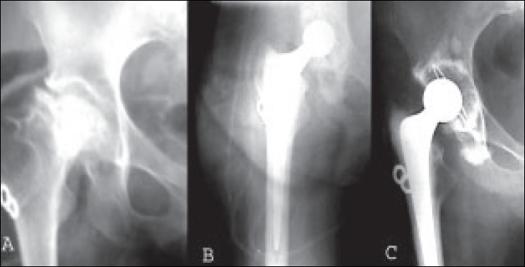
X-rays of a case of failed osteosynthesis (a) Immediate postop X-rays (b) after cemented THR. Seven years follow-up X-ray (c) showing osteolysis in Zone I and IV of femoral component and Zone I of acetabular component
